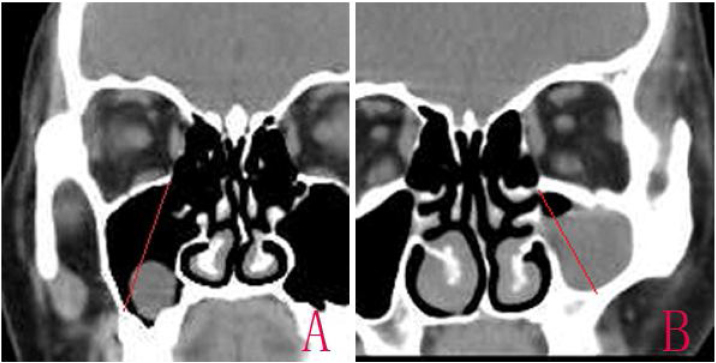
CT coronal scanning. The maxillary sinus diameter from the highest point of the superior wall to the lowest point of posterior lateral wall (A and B).
